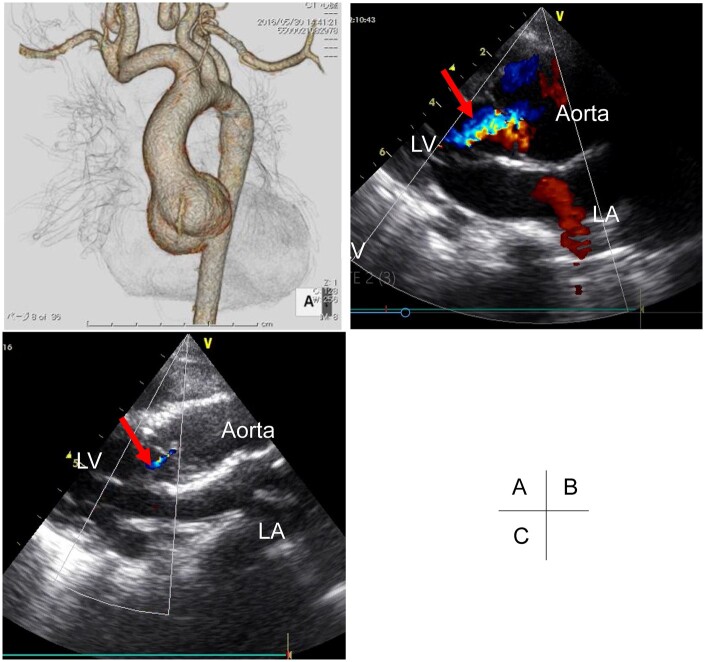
(A) Preoperative computedtomography shows dilated sinus of Valsalva. (B) Preoperative echocardiography shows moderate aortic regurgitation (red arrow) and right coronary cusp prolapse. (C) Postoperative echocardiography shows trivial aortic regurgitation (red arrow). LA: left atrium; LV: left ventricle.
